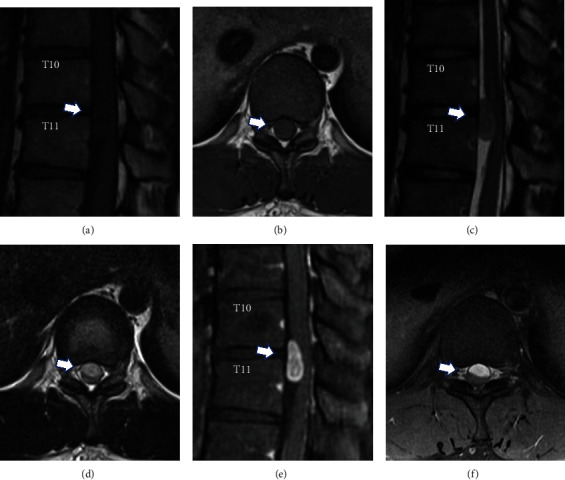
Magnetic resonance imaging of a 25-year-old man revealed an intradural extramedullary lesion at the T10 and T11 levels. The lesion shows hypointense in T1-weighted (a, b), isointense in T2-weighted (c, d) and heterogeneous in gadolinium enhancement (e, f) sagittal and axial magnetic resonance imaging of the thoracic spine. Arrows indicate the location of the lesion.
